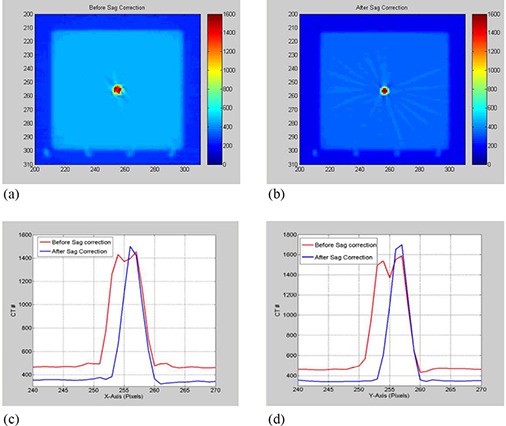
(a) and (b) show axial slices of the CBCT reconstructed from projections before and after correcting sagging shifts, respectively; (c) and (d) show line profiles from the CBCT slices through the center of the metal marker along X‐ and Y‐axis, respectively.
